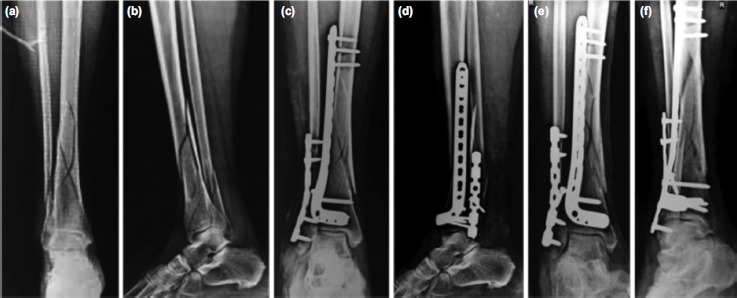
Pre-operative radiographs of intra-articular distal tibia-fibula fracture AO Type 44-C3 showing (a) antero-posterior and (b) lateral view. While, immediate post-operative radiographs showing ORIF with plating done in (c) antero-posterior view and (d) lateral view. Three months follow-up radiographs of ORIF with plating showing (c) antero-posterior and (d) lateral view.
